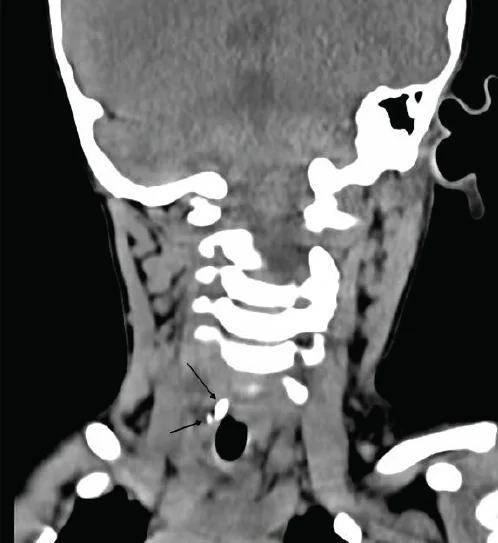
Coronal view of a non-contrast computed tomography imaging depicting glass foreign bodies (arrows).
